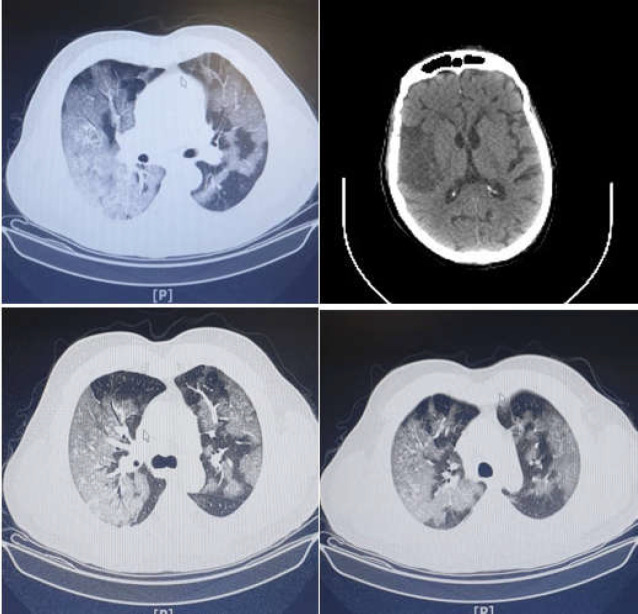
Chest and head CT scans performed during hospitalization
